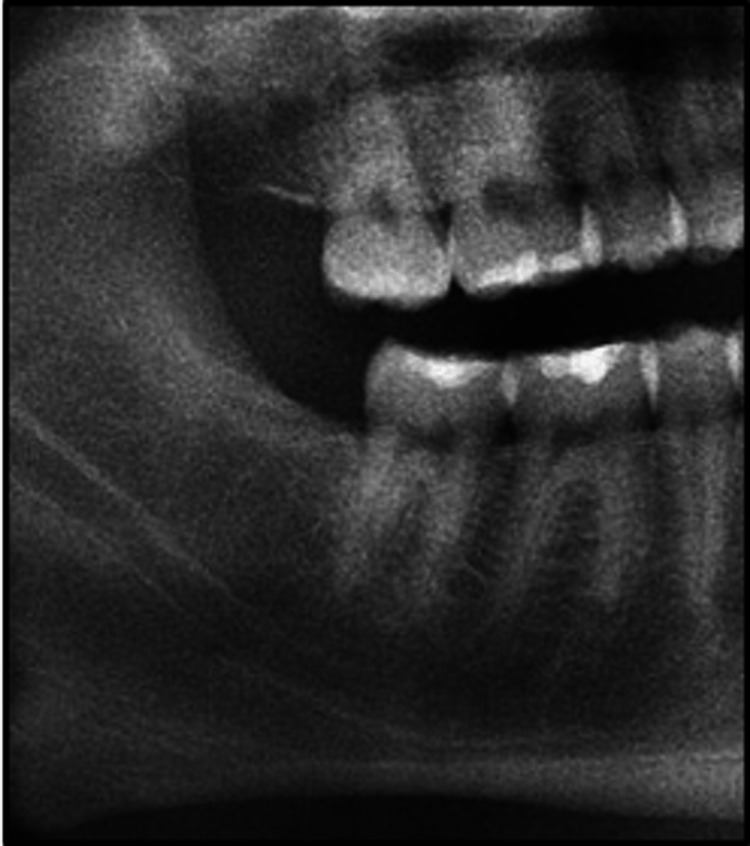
Postoperative cropped orthopantomogram at eight-month follow-up showing adequate healing
